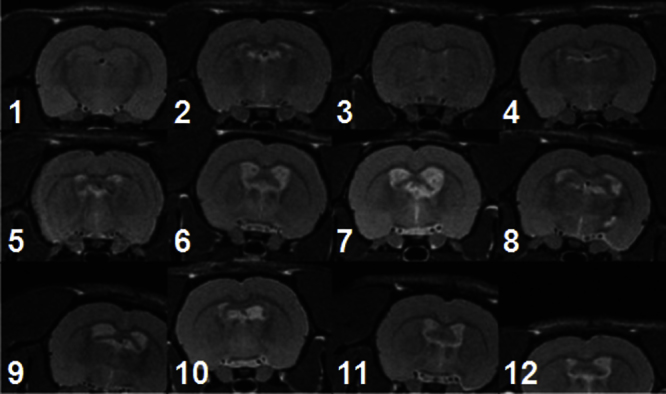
Unregistered MR images from the Parkinson's disease model cohort.
